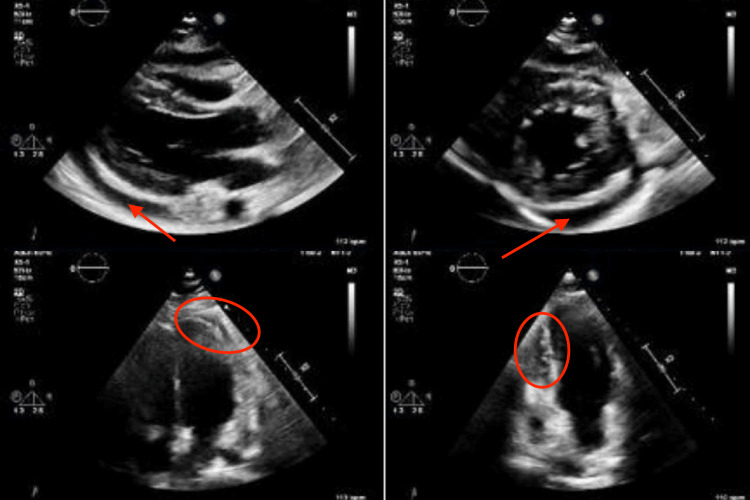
An echocardiogram showing areas of fluid collection in the pericardial cavity. The effusion is indicated by the red circles and the red arrows.
